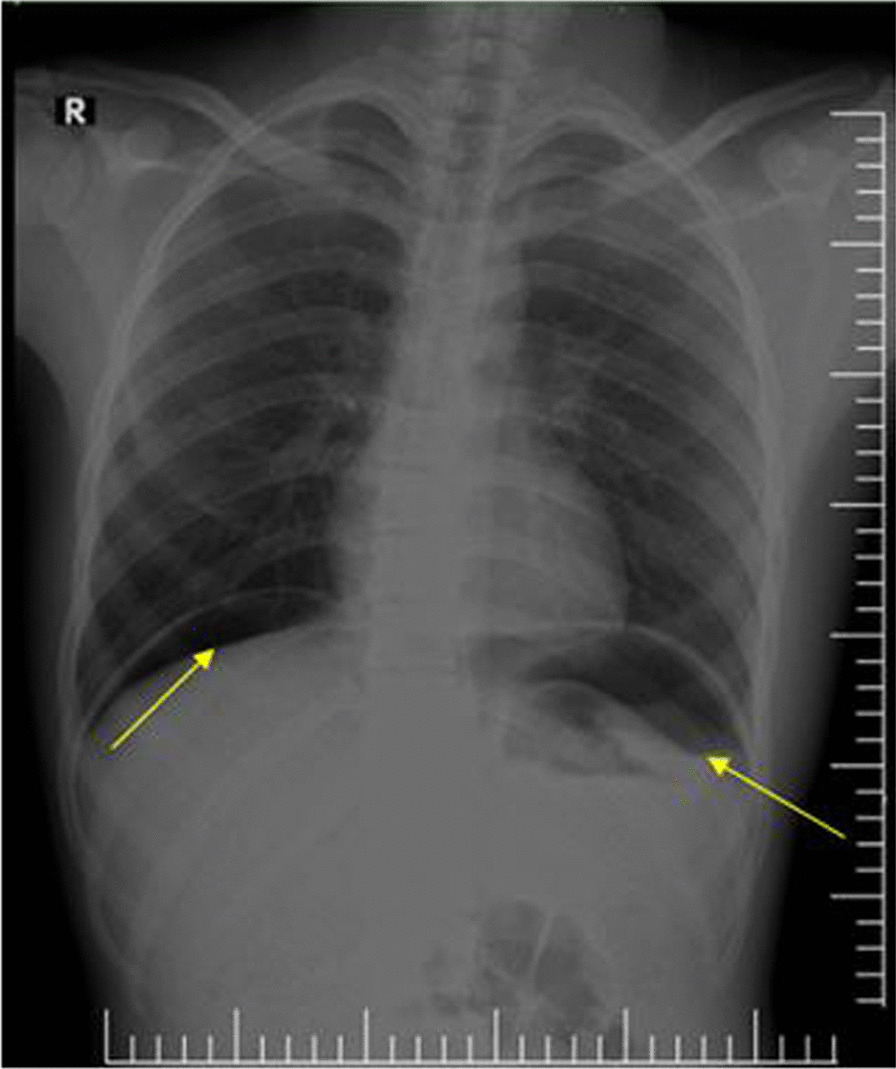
Chest X-ray showing a large volume of free sub-diaphragmatic gas with air–fluid levels under both hemidiaphragm (arrows)
